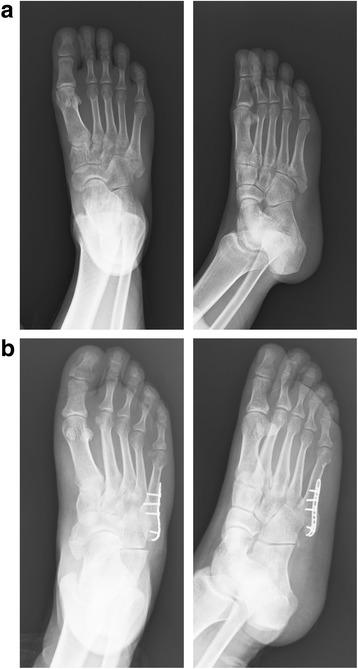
Pre- (a) and postoperative (b) radiographs of a fifth metatarsal base fracture that was treated surgically using the Locking Compression Plate (LCP) distal ulna hook plate fixation
